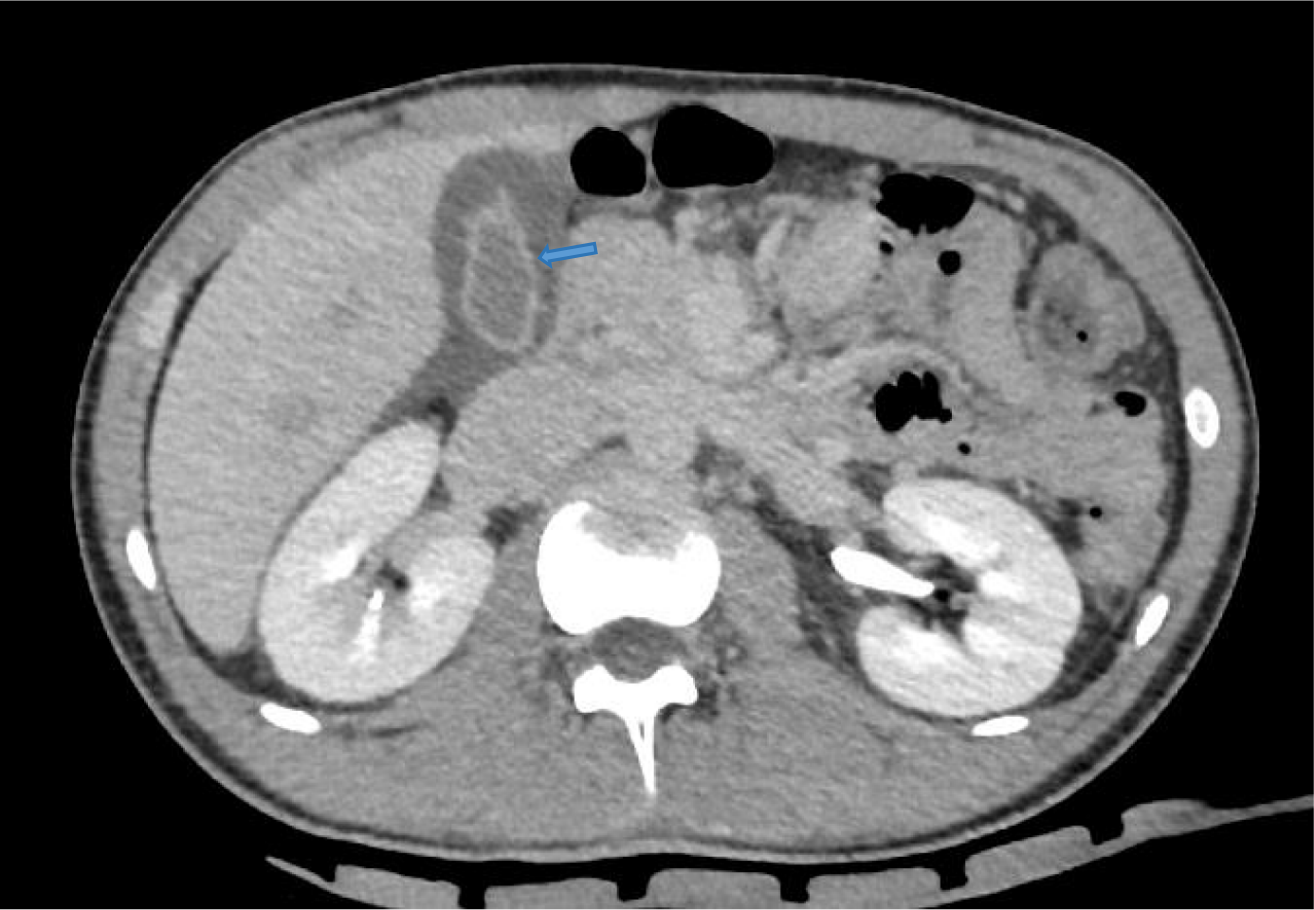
The CT scan of the abdomen revealed a thickened and edematous gallbladder wall, with no presence of gallstones, which were indicative of acalculous cholecystitis.
